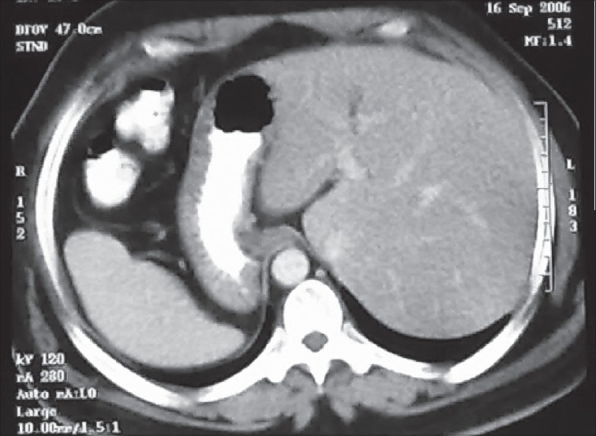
CT scan showing the transposition of major abdominal organs
